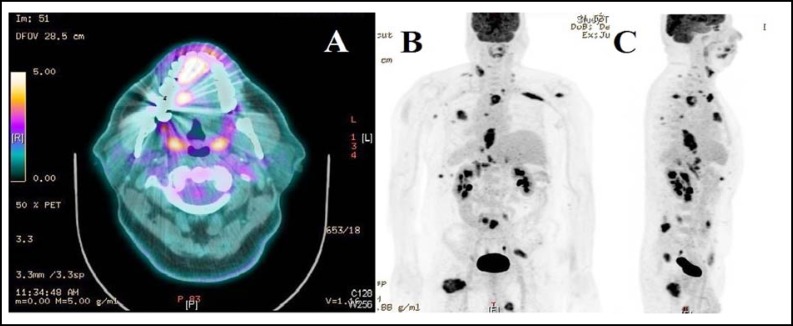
(A) 18F-FDG PET-CTaxial image showing soft tissue mass in the right lateral border of tongue showing high maximum standardized uptake value (SUVmax 5.4), (B) Topogram showing hypermetabolic metastatic disease involving supraclavicular, mediastinal, retroperitoneal lymph nodes, multiple sites of muscle involvement (SUVmax 6.1).
